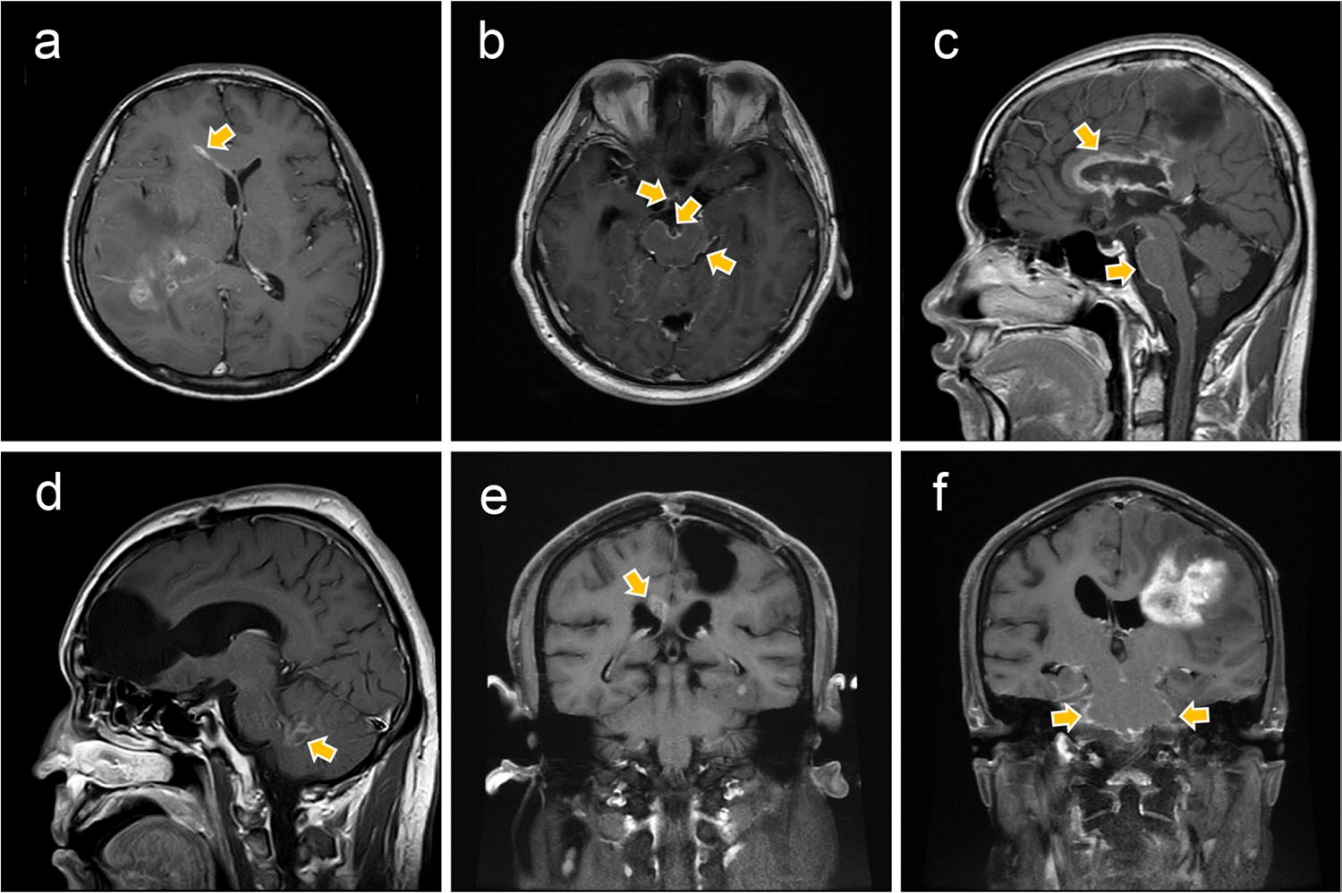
MRIs of HGG patients with leptomeningeal spread. (a) MRI type Ia: Axial T1 enhanced-contrast showed line subependymal enhancement of lateral ventricle anterior horn (yellow arrow); (b) MRI type Ib: Axial T1 enhanced-contrast showed line leptomeningeal enhancement around midbrain and optic chiasma (yellow arrows); (c) MRI type II; Sagittal T1 enhanced-contrast images showed subependymal enhancement along the corpus collosum, line leptomeningeal enhancement in the surface of the brain stem and nodular enhancement in cerebellar tonsil (yellow arrows); (d) Nodular LMS: Sagittal T1 enhanced-contrast images showed nodular LMS at the bottom of the fourth ventricle was secondary to frontal lobe glioma (yellow arrow); (e) Coronal T1 enhanced-contrast MRI showed stable disease at initial tumor site and contralateral subependymal enhancement (yellow arrow); (f) Coronal T1 enhanced-contrast MRI showed local recurrence and leptomeningeal enhancement around brain stem (yellow arrows)
